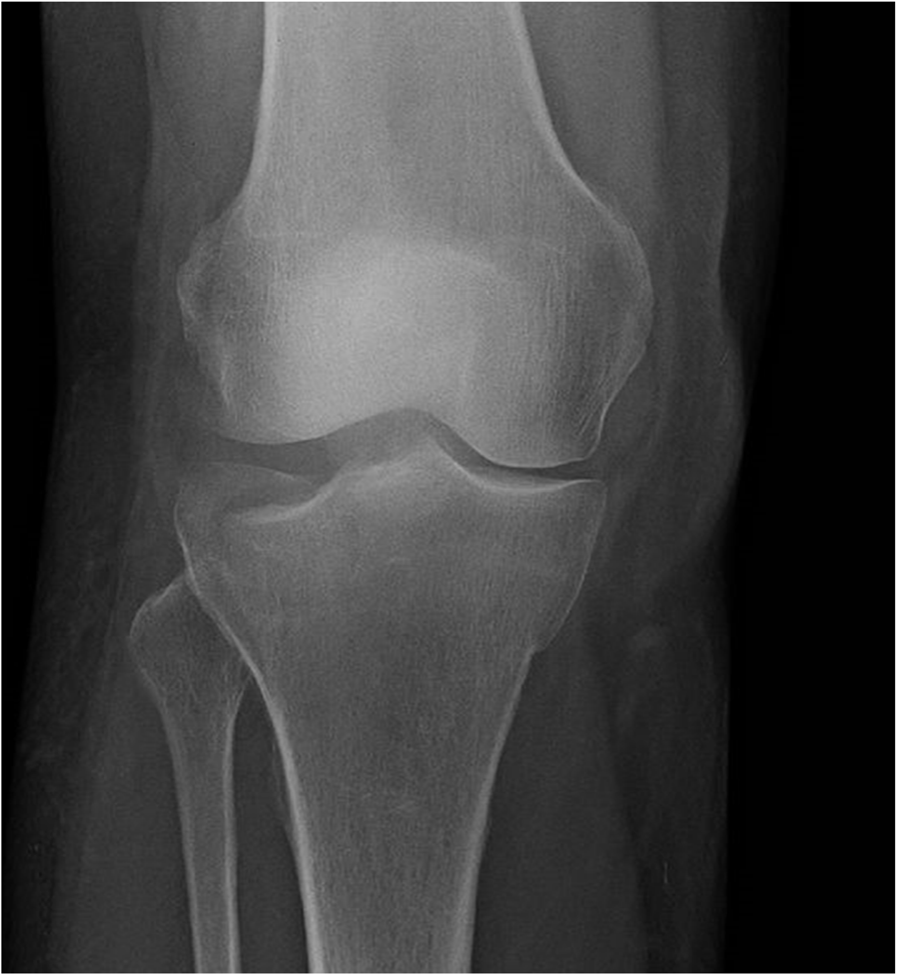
X-ray imaging of a lateral tibial plateau fracture. From https://commons.wikimedia.org/wiki/File:Tibial_Plateau_Fracture.jpg
